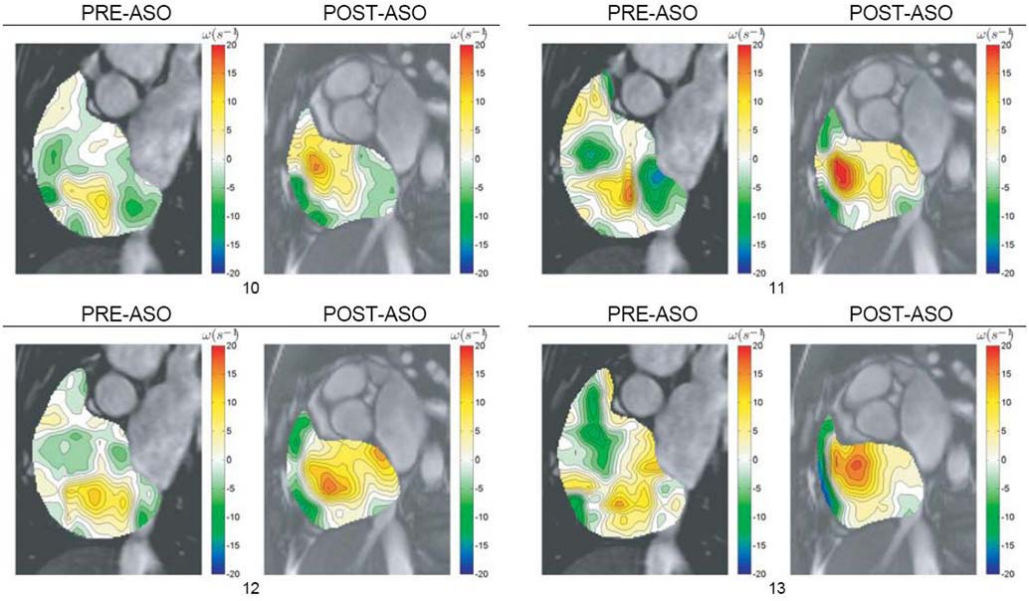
Vorticity visualization of right atrial flow pre- and post-ASO. Vorticity contour maps are superimposed onto cardiac MR images and presented. The vorticity representation by the flow map in color codes can be referenced against scales (in metric units of per second) on the right hand side. The range of the vorticity given by *ω*
